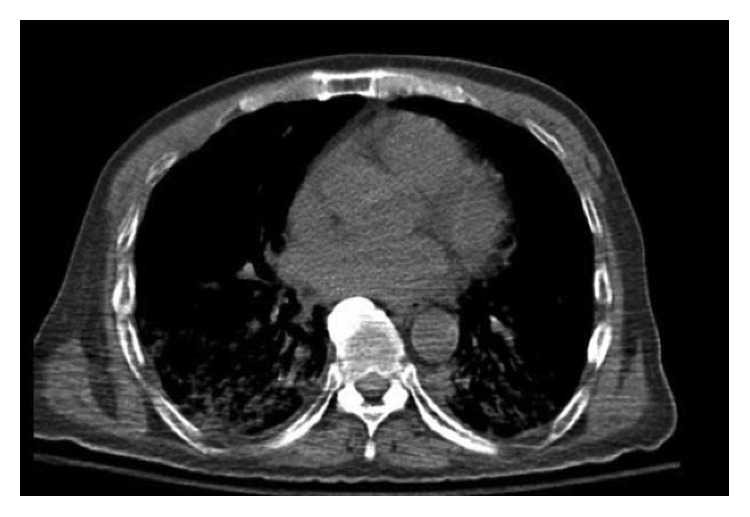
A repeat chest CT showed resolution of previously well-defined areas of perihilar ground-glass opacities but development of hazy areas of ground-glass opacification throughout both lungs with more confluent abnormalities in bilateral lower lobes.
